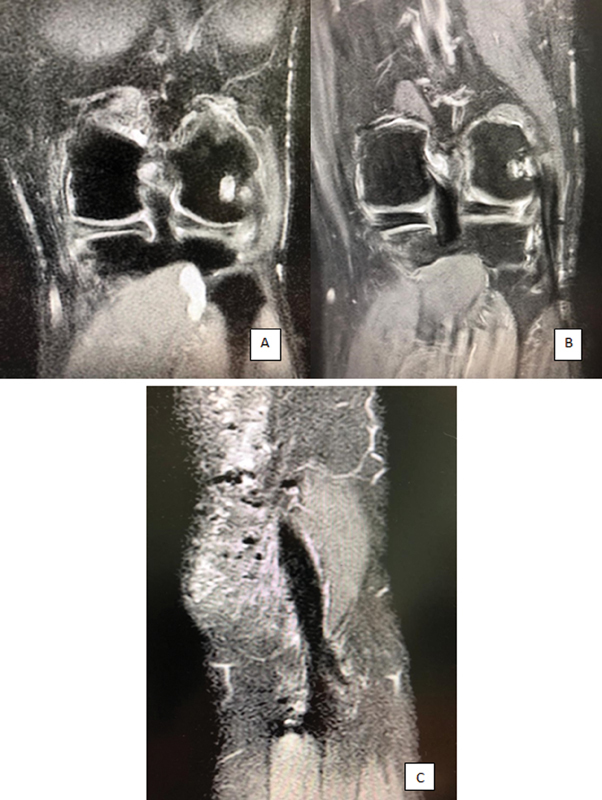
Preoperative magnetic resonance imaging (coronal)
